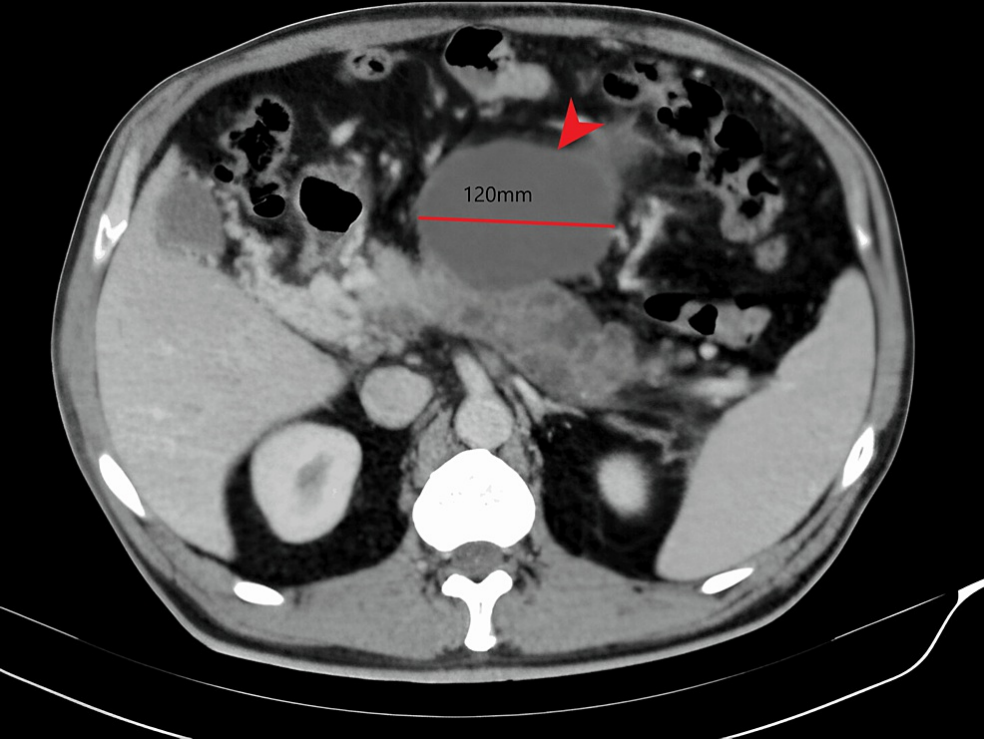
Initial CT scan It shows portal thrombosis right and left branches in the hepatic hilum, probably secondary to thrombosis during the time of evolution. In the pancreas, two well-defined anechoic structures are identified with echogenic content in its interior of 12 cm and another of 9 cm suggestive of pancreatic pseudocysts that prevent the complete visualization of the gland. The body of the pancreas is also partially visualized with multiple chiascopic images between 12 and 15 mm.
